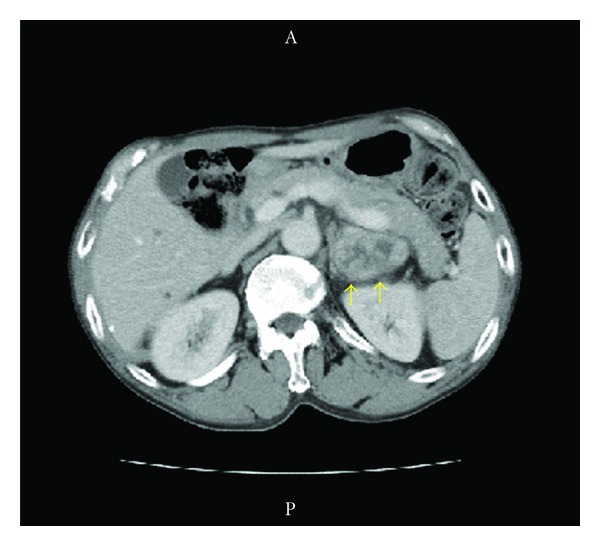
Computed tomography at 8 months after cystectomy shows a left adrenal tumor (5.0 × 4.0 cm).
